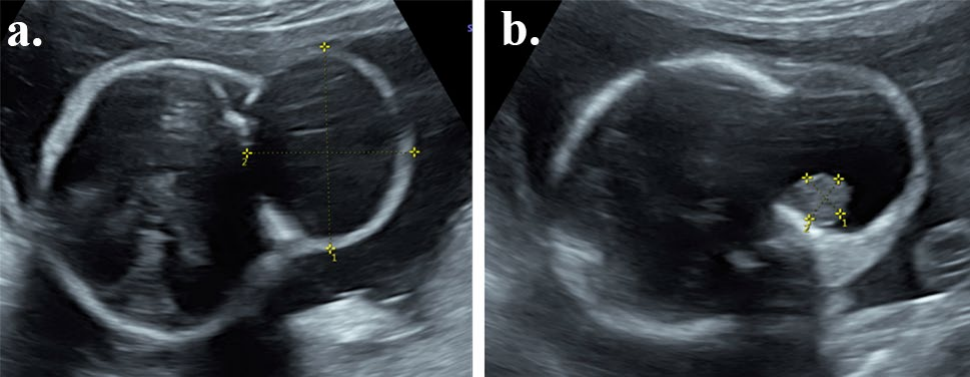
Ultrasound at 22 weeks’ gestation. a and b are ultrasound images of the lesion at 22 weeks gestation. The lesion (a) measures 4 × 3.5 × 3 cm in size with a single solid component of 1.02 × 1.03 cm size seen in (b)
